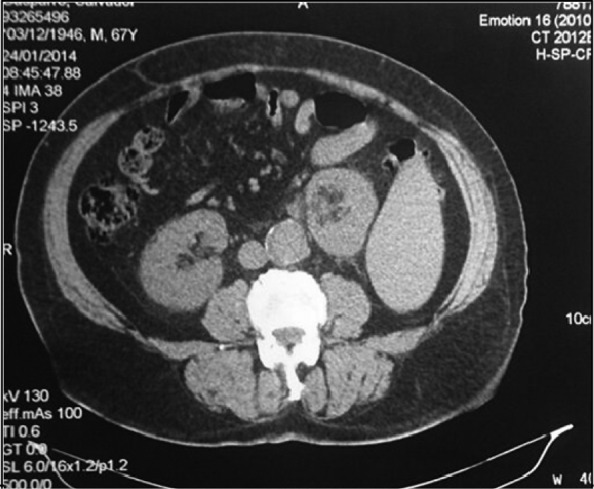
Abdominal CT that evidences splenomegaly and displacement of the left kidney.
